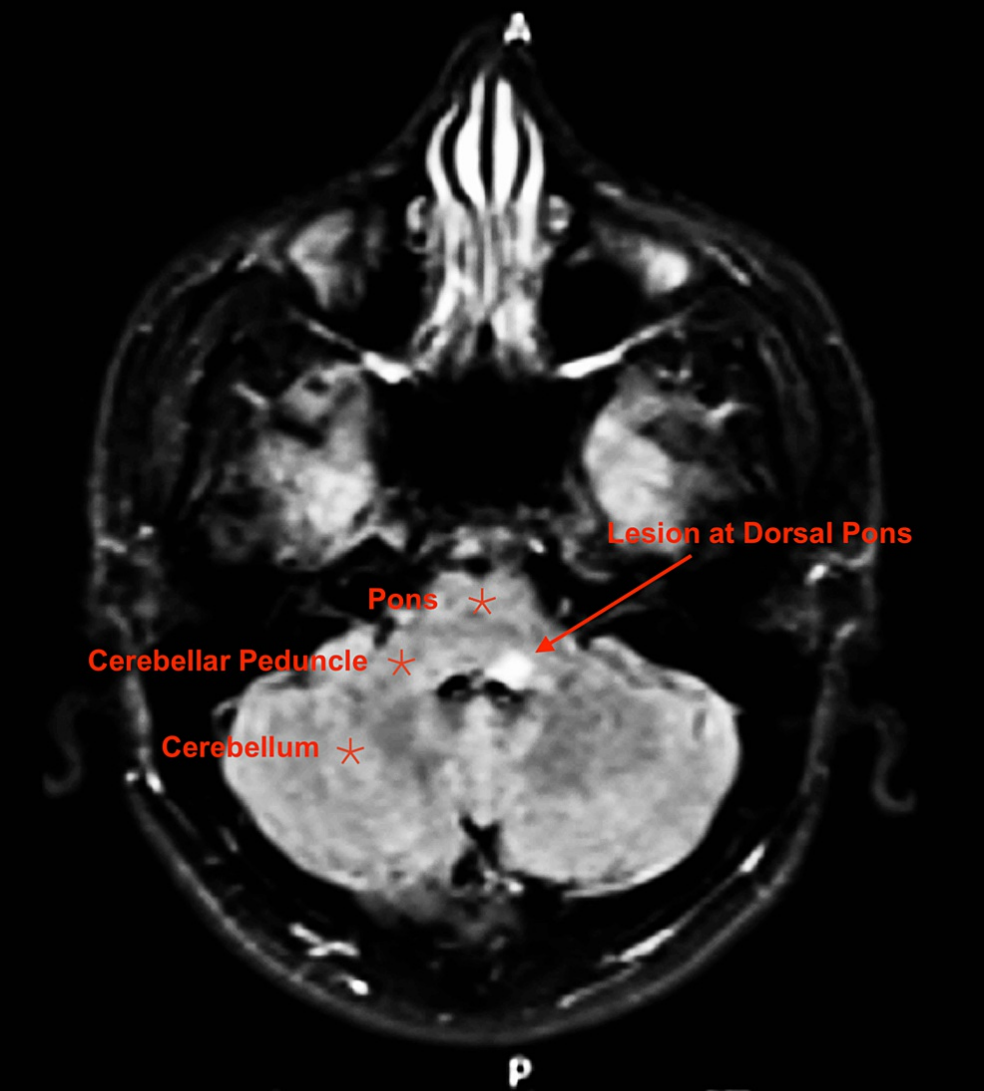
MRI brain FLAIR-weighted image in axial view Axial view MRI brain FLAIR-weighted image displaying a hyperintense lesion situated at the level of the middle cerebellar peduncle. The lesion is depicted as a bright area against the surrounding tissue, suggestive of a potential pathology such as inflammation or demyelination MRI: magnetic resonance imaging; FLAIR: fluid-attenuated inversion recovery
